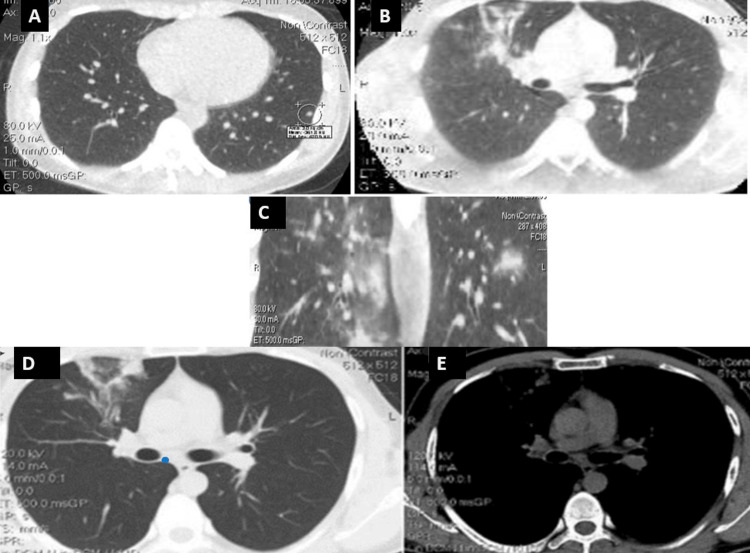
High-quality diagnostic imaging with modified low-dose protocols. (A,B,C,D) Lung window screening and (E) mediastinal window. These types of protocols are successfully used for repeat CT scans and hospitalization monitoring.
